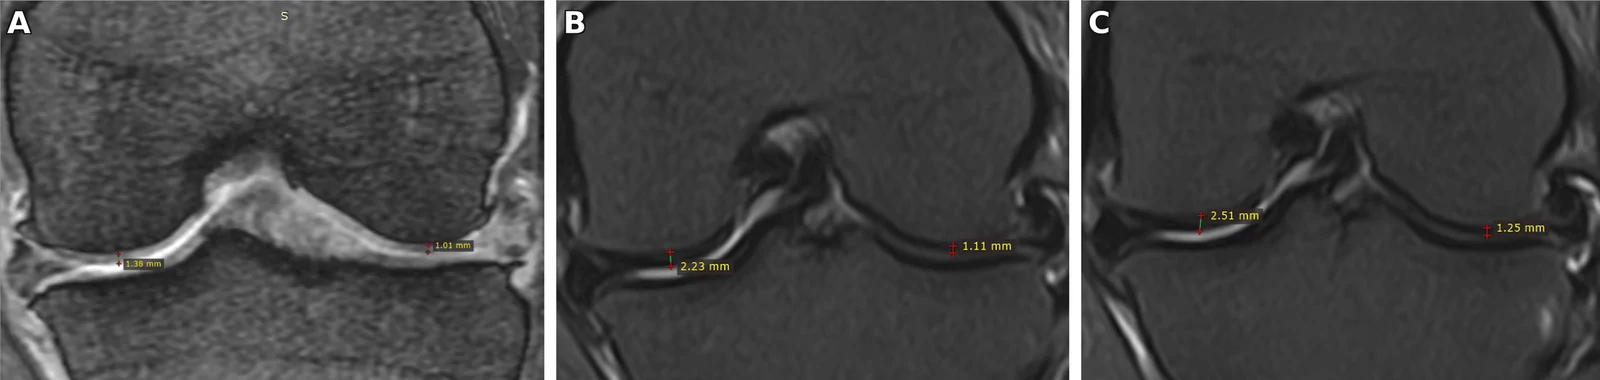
Serial coronal MRI of the left knee showing tibiofemoral cartilage-thickness measurements Serial coronal MRI of the left knee showing tibiofemoral cartilage-thickness measurements. (A) Day -52; (B) Day 78; (C) Day 407. Cartilage thickness increased at the two measured points (1.38 to 2.23 to 2.51 mm and 1.01 to 1.11 to 1.25 mm). Panel A was acquired on a different scanner without a T2 STIR sequence. Cartilage thickness was measured using the anterior cruciate ligament as the anatomical landmark to approximate the same location across time points; measurements were made by a single, non-blinded assessor without formal reliability testing. STIR, short tau inversion recovery
